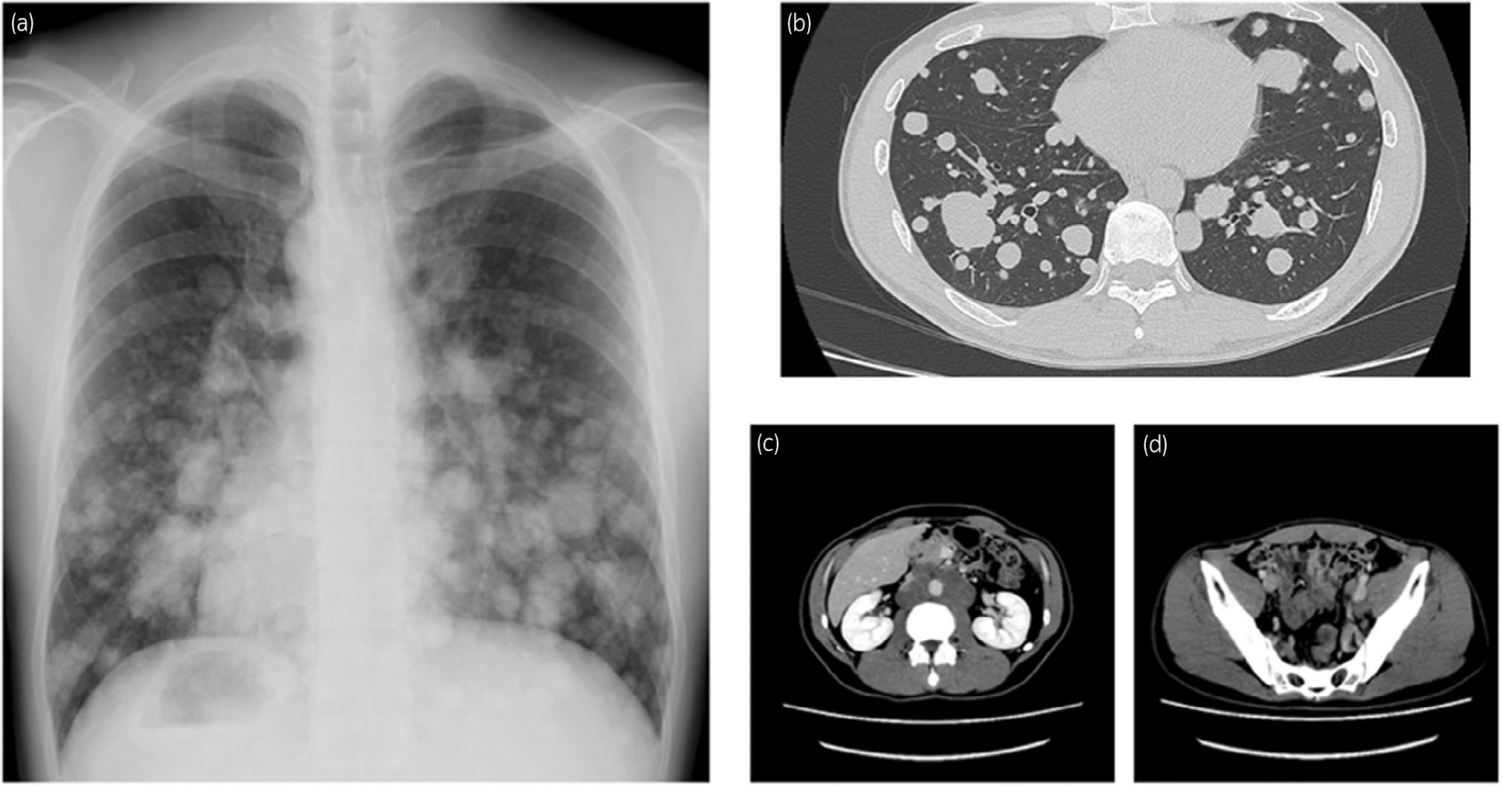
Before BEP, radiography and CT imaging findings. (a) Chest radiography: multiple nodules in both lungs. (b) Multiple lung metastases. (c) Hilar lymph and paravalvular lymph node metastases. (d) Left pelvic lymph node metastases.
